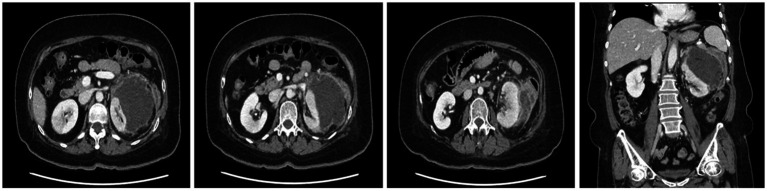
Follow-up CT showing an approximately 11 cm perinephric abscess with peripheral enhancement, medial displacement of the left kidney, and adjacent edematous changes in the descending colon.
